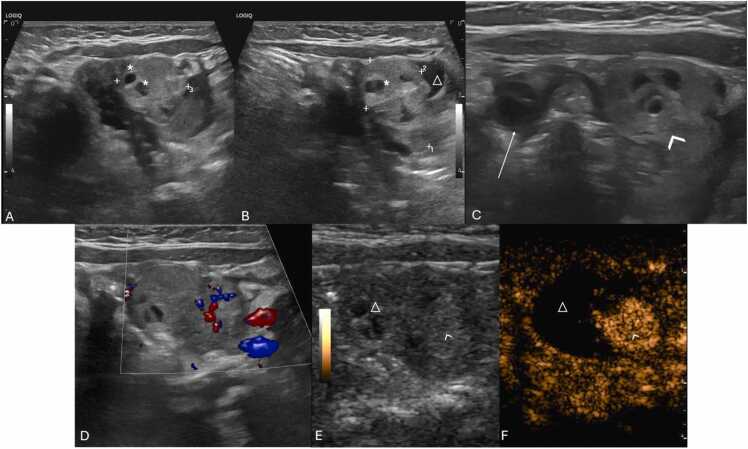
(A–F) Surgically confirmed ovarian torsion in a 4-month-old infant, with irritability and abdominal discomfort referred by the mother. Transabdominal pelvic ultrasound over the hypogastrium, revealed an asymmetrically enlarged, hyperechoic and inhomogeneous left ovary (between calipers in A and B) and containing several follicles (* in A and B). Notice the presence of free fluid around the left ovary (△ in B). Compare the difference in size and appearance between the right (arrow in C) and left ovaries (arrowhead in C). Doppler US of the left ovary (D) revealed absent vascular flow in the ovarian parenchyma, with preserved flow in what appeared to be a torsed vascular pedicle - raising suspicion for ovarian torsion. To confirm the diagnosis, CEUS was performed, showing no enhancement in the left ovary (△ in E and F) while maintaining normal enhancement of the associated vascular pedicle (arrowhead in E and F). Intraoperative findings confirmed an ischemic left ovary.
